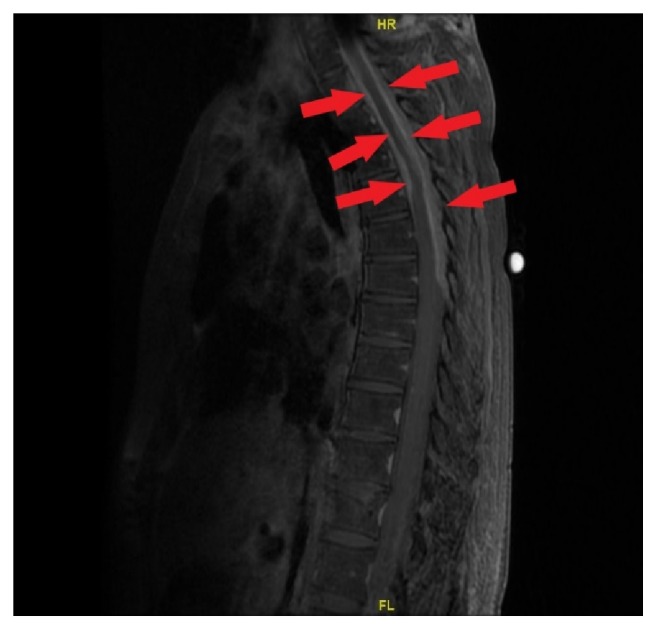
T1-weighted Thoracic spine Magnetic Resonance Imaging showing diffuse extradural mass effect and enhancement from the T1 level to the T5 level anteriorly and to the T7 level posteriorly; most prominent at the T4-T5 level where there is marked spinal canal stenosis and mass effect upon the spinal cord.
